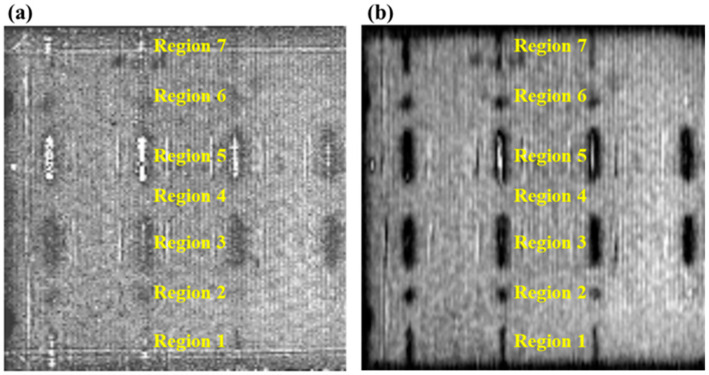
Laser ultrasonic C-scan results: (a) original image with raw data and (b) improved image with SAFT.
